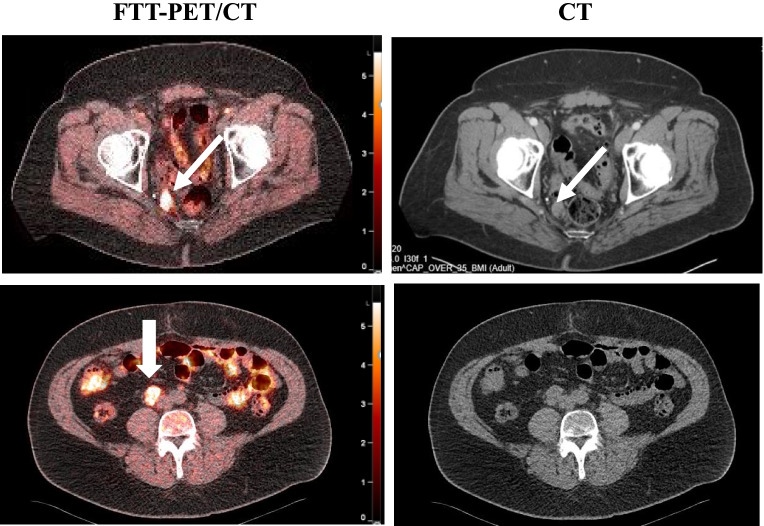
Prostate adenocarcinoma (#4). Fused FTT-PET/CT images (left) and CT images (right). There are FTT + right perirectal (top row) and right internal iliac lymph nodes (bottom row arrows). The scale bar on PET images: SUV_max_ = 5.5
